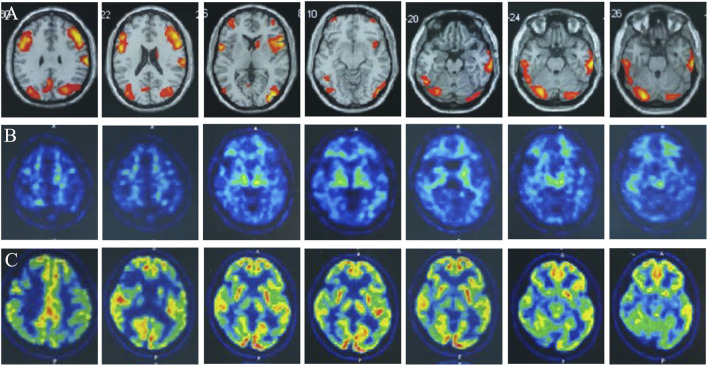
Multimodality molecular imaging of the patient: (A) Multimodal molecular imaging of 18-fluoro-2-deoxy-D-glucose positron emission tomography (^18^F-FDG PET-CT) results suggested hypometabolism in dorsolateral frontal cortex, dorsolateral parietal cortex, bilateral precuneus, lateral of right temporal lobe and right occipital lobe, and right caudate nucleus. (B) 11-carbon-Pittsburgh positron emission tomography (^11^C-PIB PET-CT) showed no amyloid plaque deposition in the brain. (C) 18-fluoro-S16-positron emission tomography (^18^F-Flortaucipir-tau PET-CT) showed tau deposition in bilateral thalamus, caudate nucleus, midbrain, pons, and bilateral medial temporal lobe.
